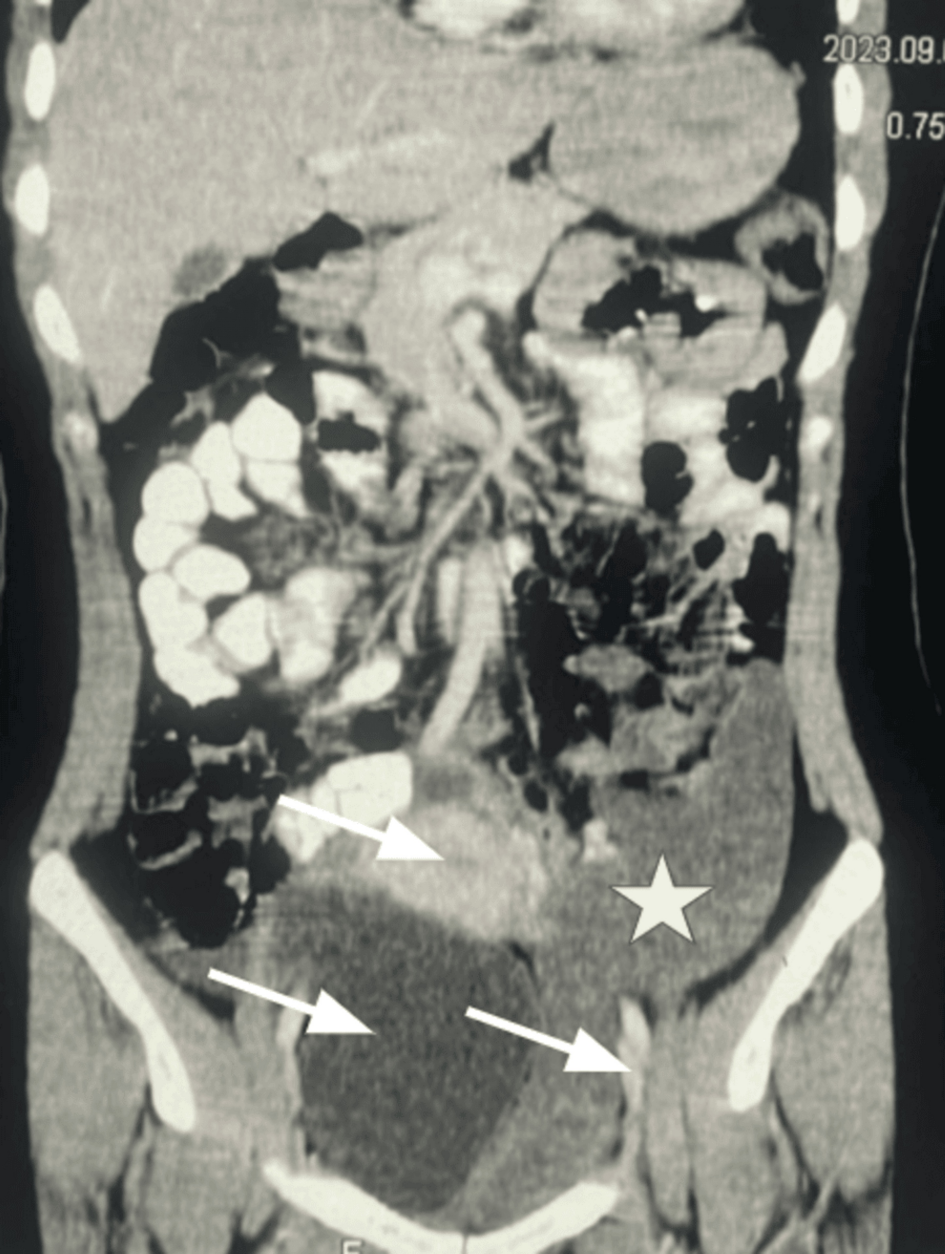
Contrast-enhanced CT of the abdomen showing a large, minimally enhancing soft tissue lesion occupying the left pelvis, with anterior displacement of the uterus and compression of surrounding pelvic structures. Star marks the center of the mass while arrow points to areas of interest.
